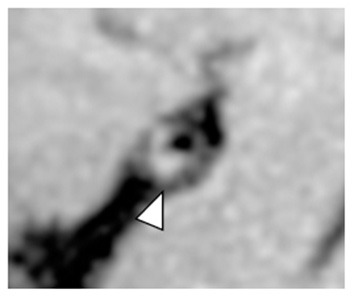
Intrinsic T1 hyperintense signal of intracranial plaque on precontrast VW-MRI. Orthogonal view of a precontrast VW-MRI of the middle cerebral artery shows intrinsic T1 hyperintense signal (arrowhead) and positive (outward) wall remodeling (arrowhead).
